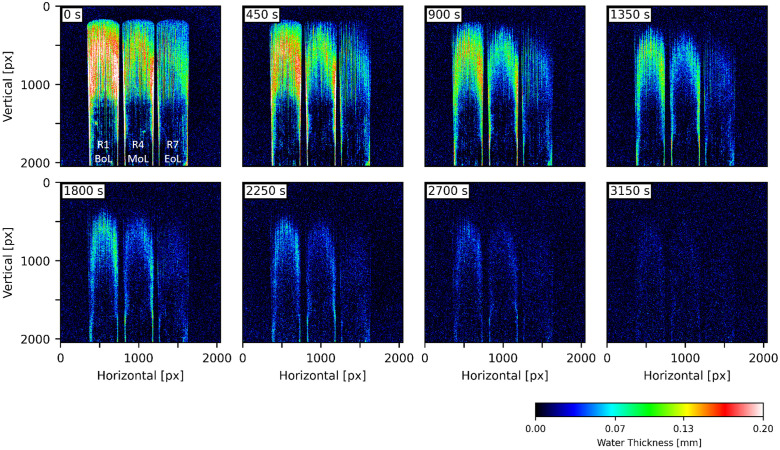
Water thickness images derived from in situ neutron radiography of 40-min-wet reeds drying. Three reeds are R1 (BoL), R4 (MoL), and R7 (EoL) from left to right in each timestamp. The reeds were immersed in water for 40 min before imaging.
